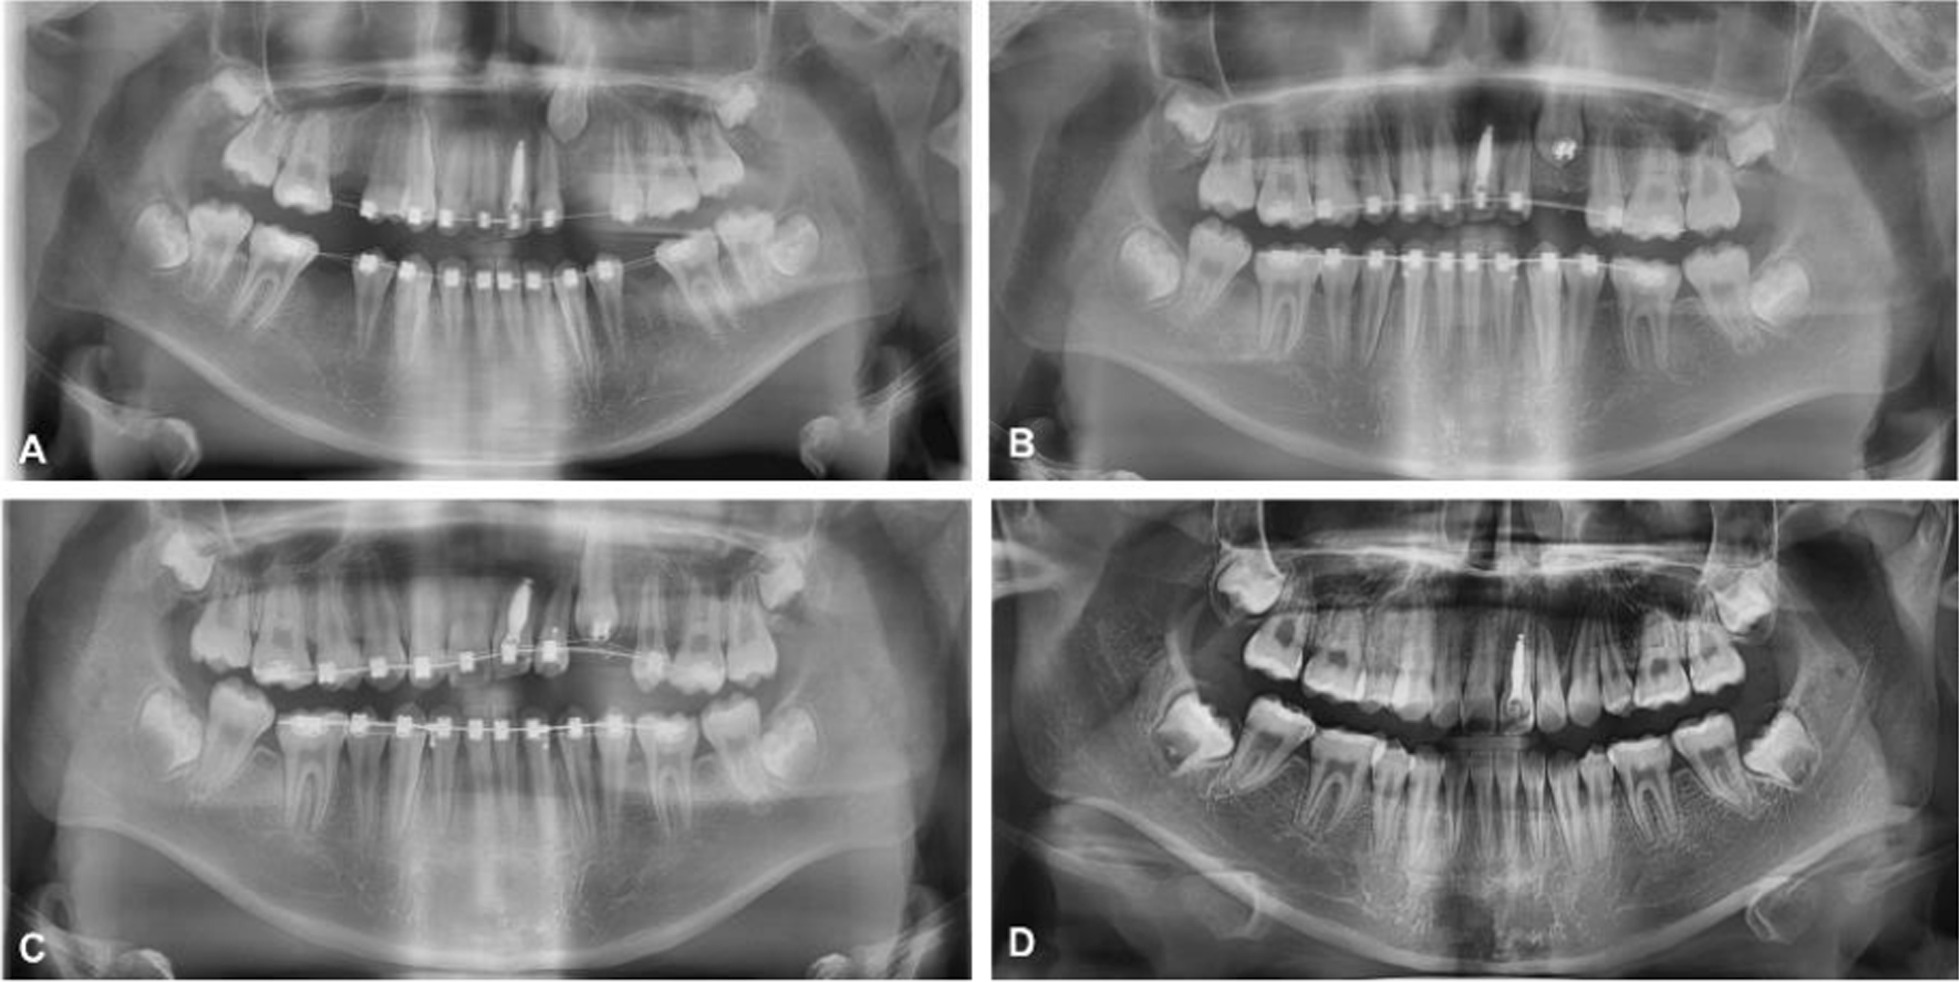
Orthopantomograms during orthodontic treatment (A–D)
